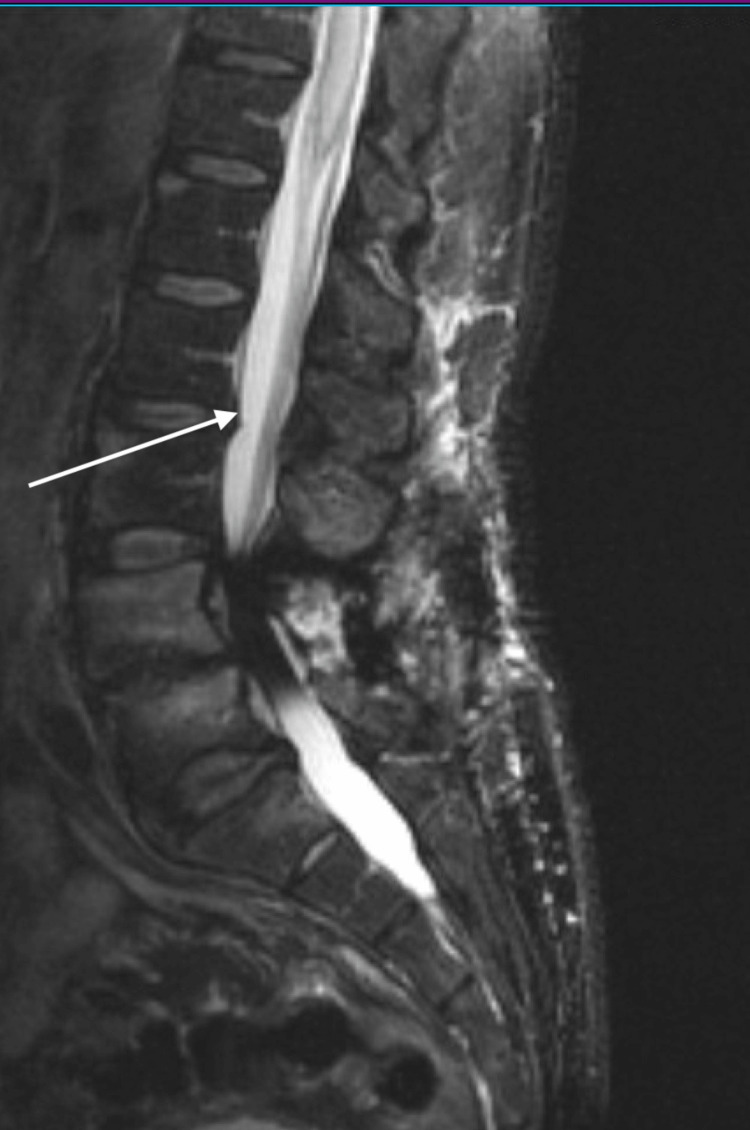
Magnetic resonance imaging of the lumbar spine showing abnormal hyperintensity from the T9 segment to the conus
